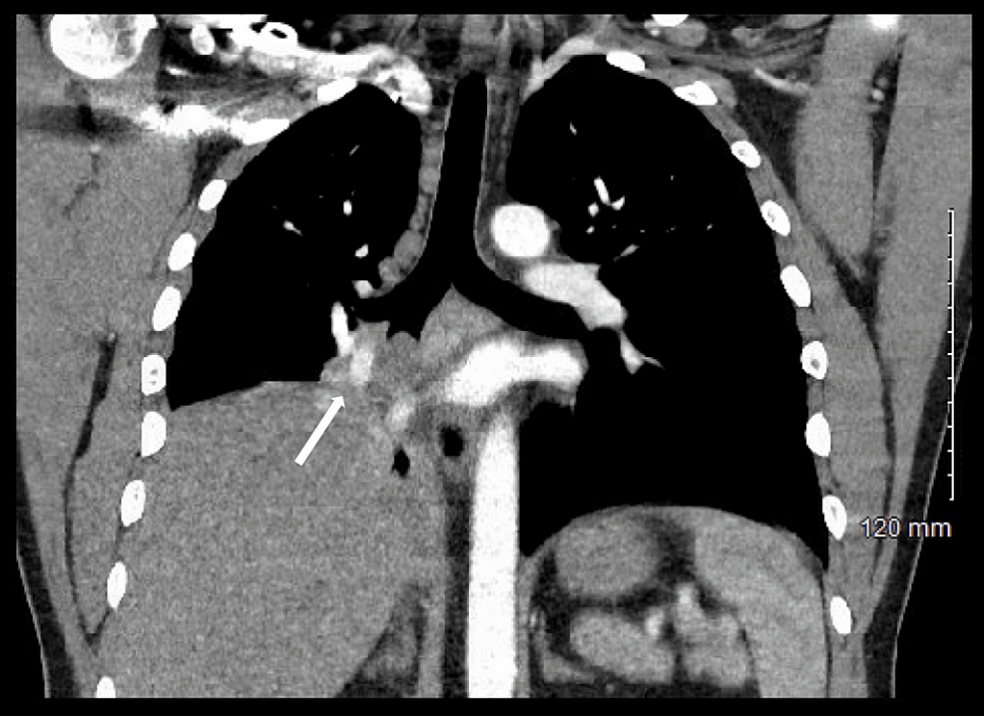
Coronal view of computed tomography angiography of the chest with contrast per the pulmonary embolism protocol, demonstrating persistent soft tissue density at the right hilum
